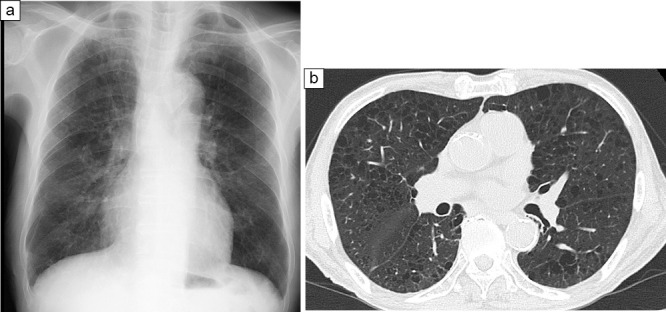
Imaging study 2 months after admission: a: Plain chest X-ray (standing position). b: Chest CT scan. a, b: No pleural effusion was observed on either side.
